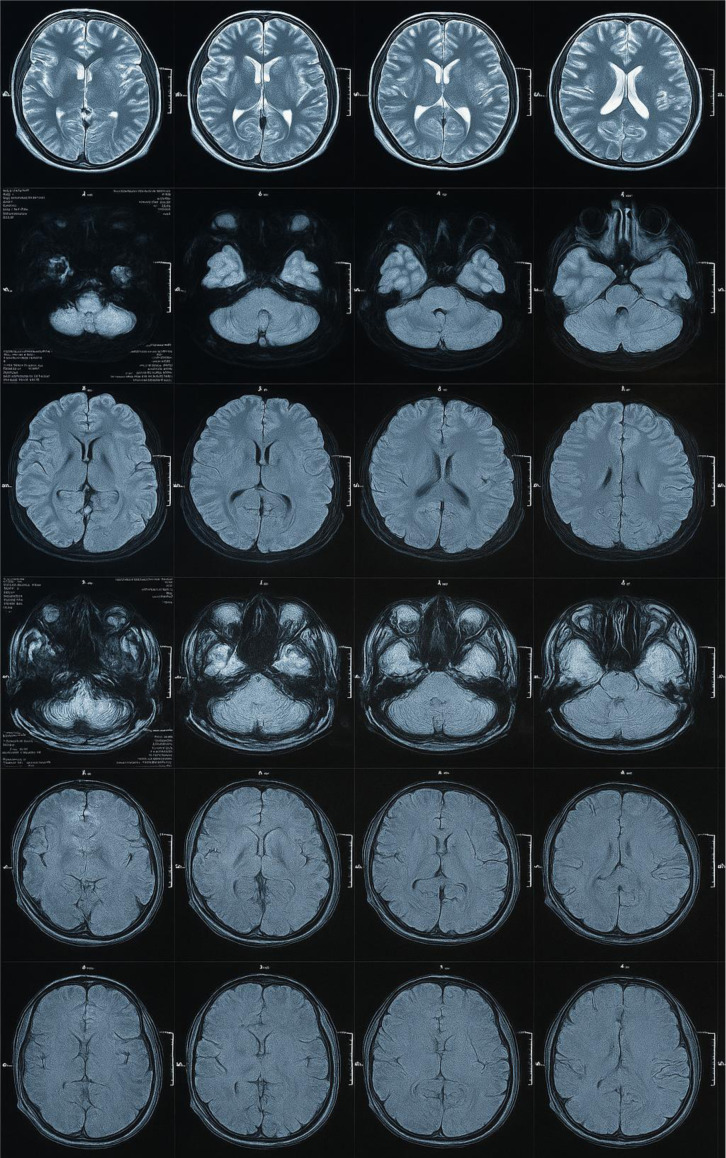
MRI Brain (FLAIR and T2 Axial Sequences) in a Case of Scrub Typhus Encephalitis. MRI brain axial sequences (FLAIR and T2-weighted images) showing suggestive findings of scrub typhus encephalitis - the scans reveal bilateral, symmetric hyperintense lesions predominantly involving the thalami, basal ganglia, and brainstem-especially the midbrain and pons. Subtle involvement of the cerebellar peduncles and periventricular white matter is also noted. No significant mass effect, midline shift, or hemorrhagic transformation is present.
